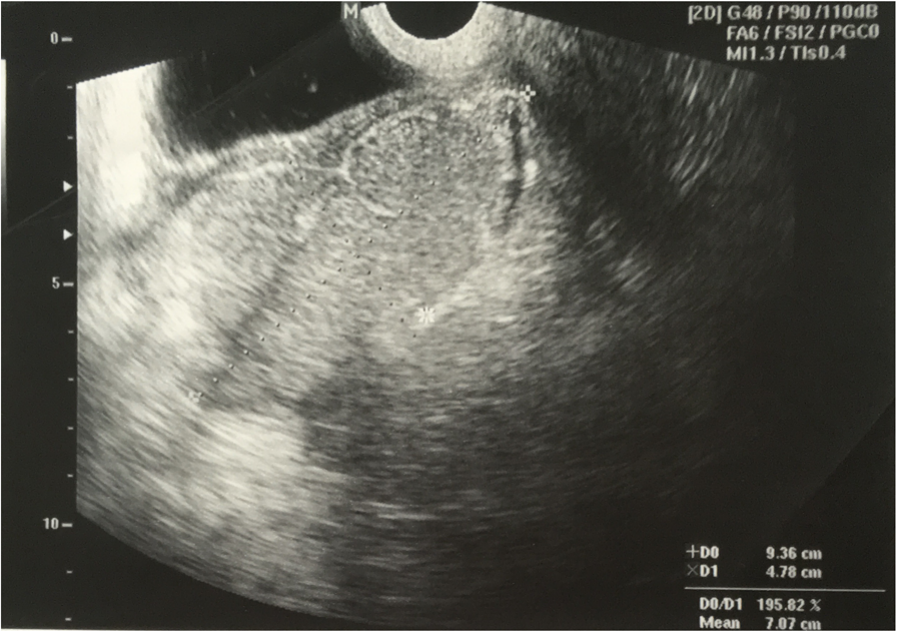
Transvaginal ultrasonography demonstrating a large left tubo-ovarian abscess size 9.4 × 4.8 cm located at anterior of the uterus
